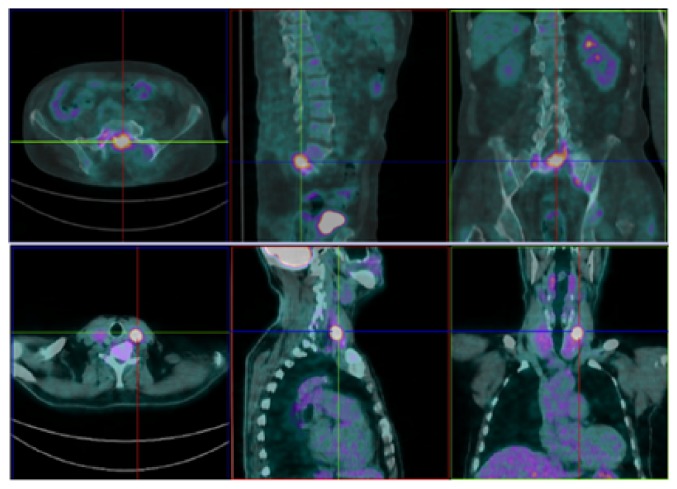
18F-FDG-PET showing uptake of the radiopharmaceutical drug in thyroid left lobe, L5 vertebrae, and pelvic bones [Case 1].
